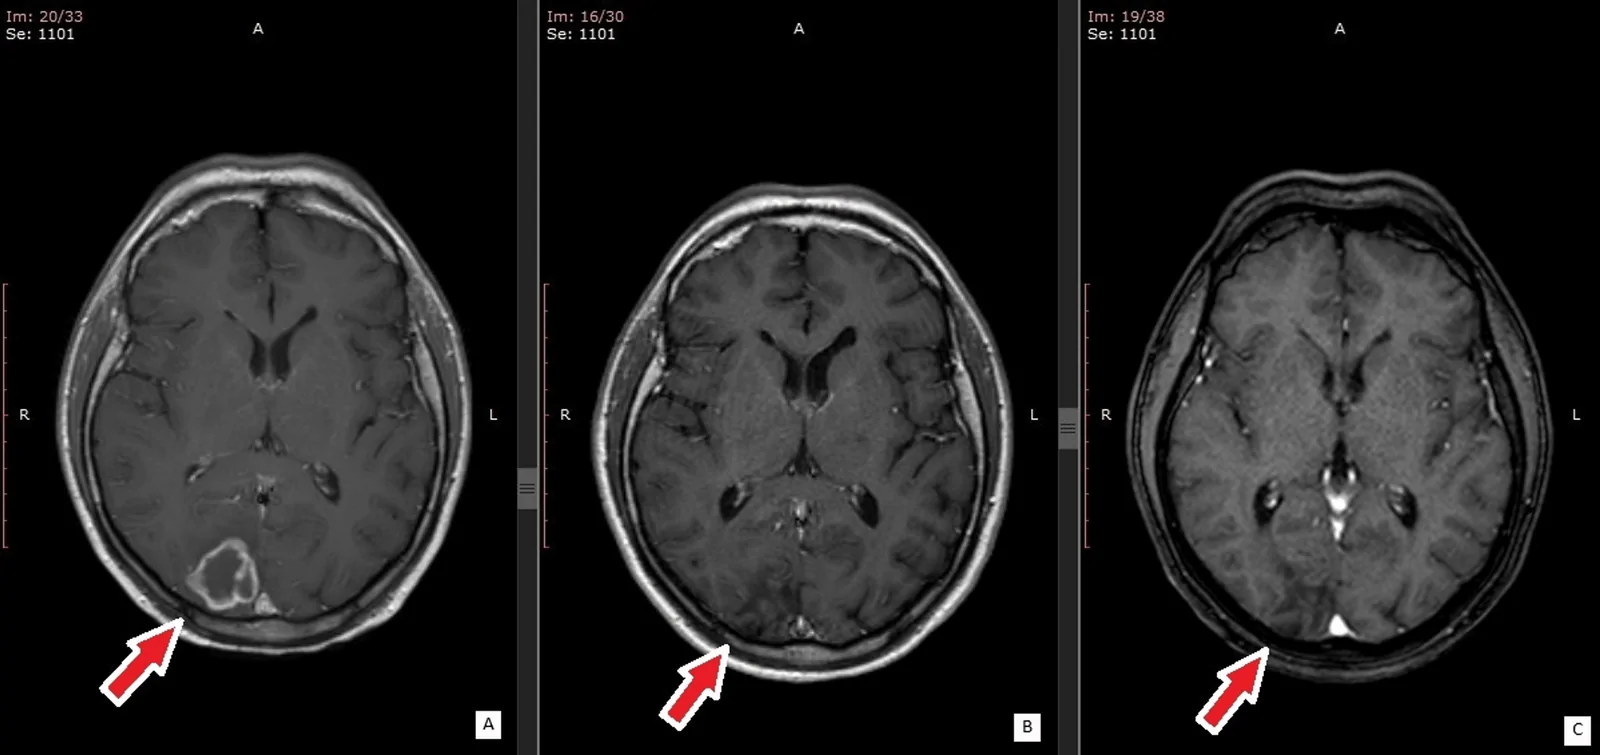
Comparative MRI. The images show post-RT follow-up in the contrast-enhanced T1 phase: from left to right, December 2024 (A), July 2025 (B), and February 2026 (C).
